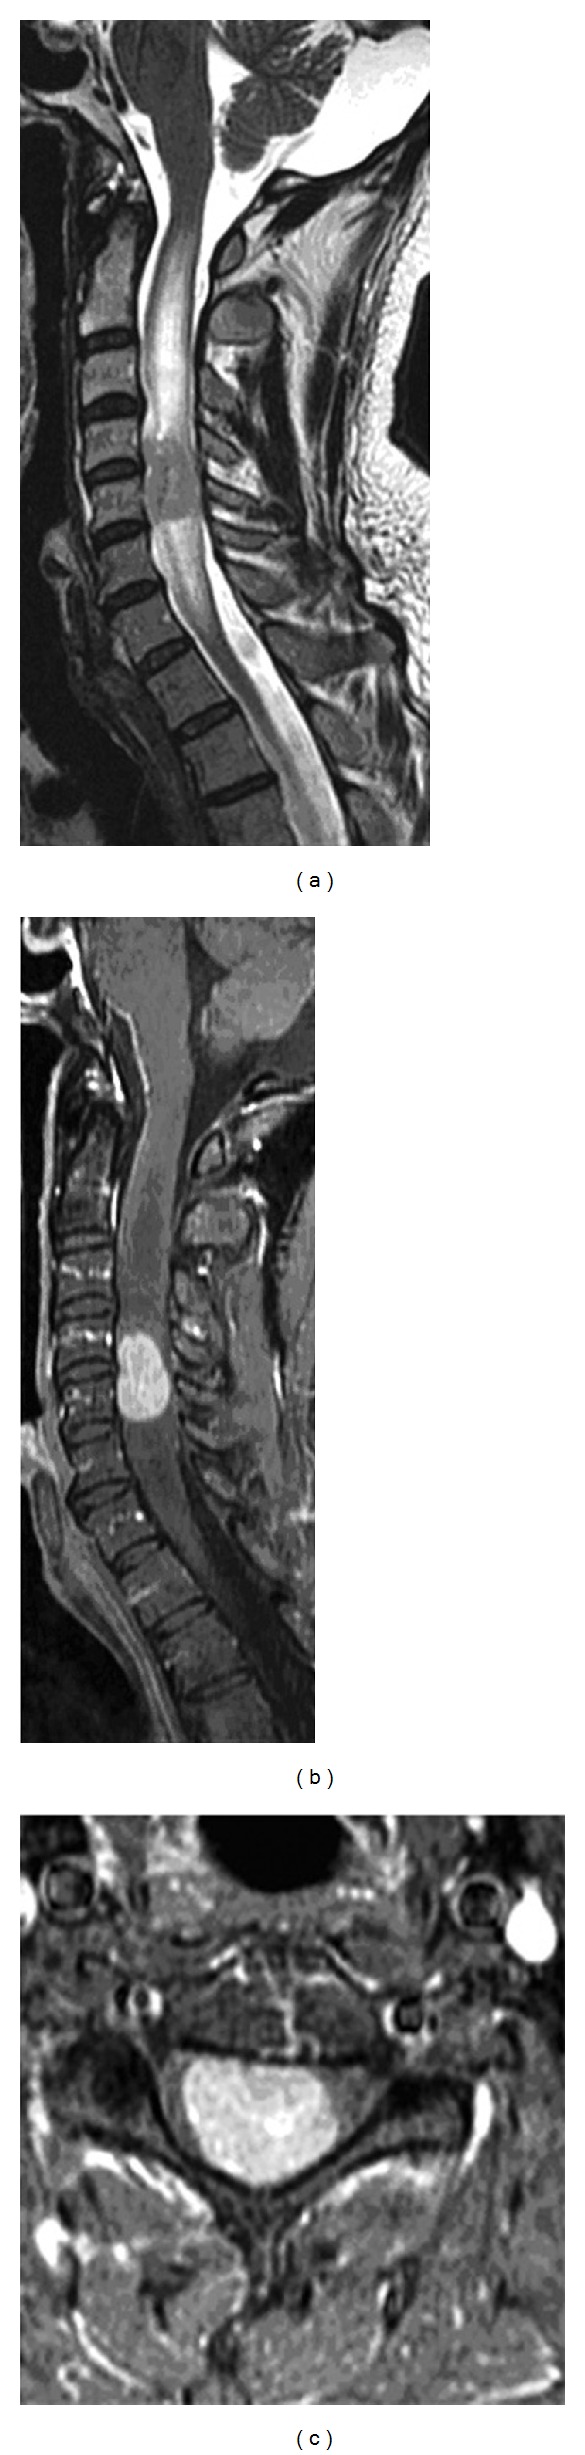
(a) Sagittal T2-weighted image of the cervical spine with an isointense lesion at C4-5 with surrounding T2 hyperintensity suggestive of edema. (b) Sagittal T1-weighted image with gadolinium enhancement showing an avidly enhancing lesion with surrounding cord edema. (c) Axial T1-weighted image with gadolinium enhancement revealing an intramedullary homogenously enhancing lesion with severe cord compression.
